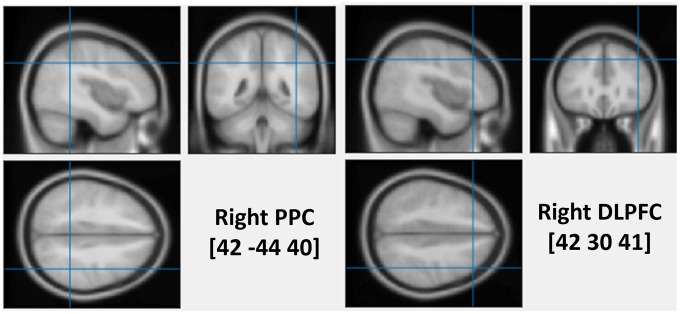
The average stimulated rPPC (MNI: 42, –44, 40) and rDLPFC (MNI: 42, 30, 41) sites. The position was verified using individual MRI scan co-registered to their head landmarks using BrainSight software.
